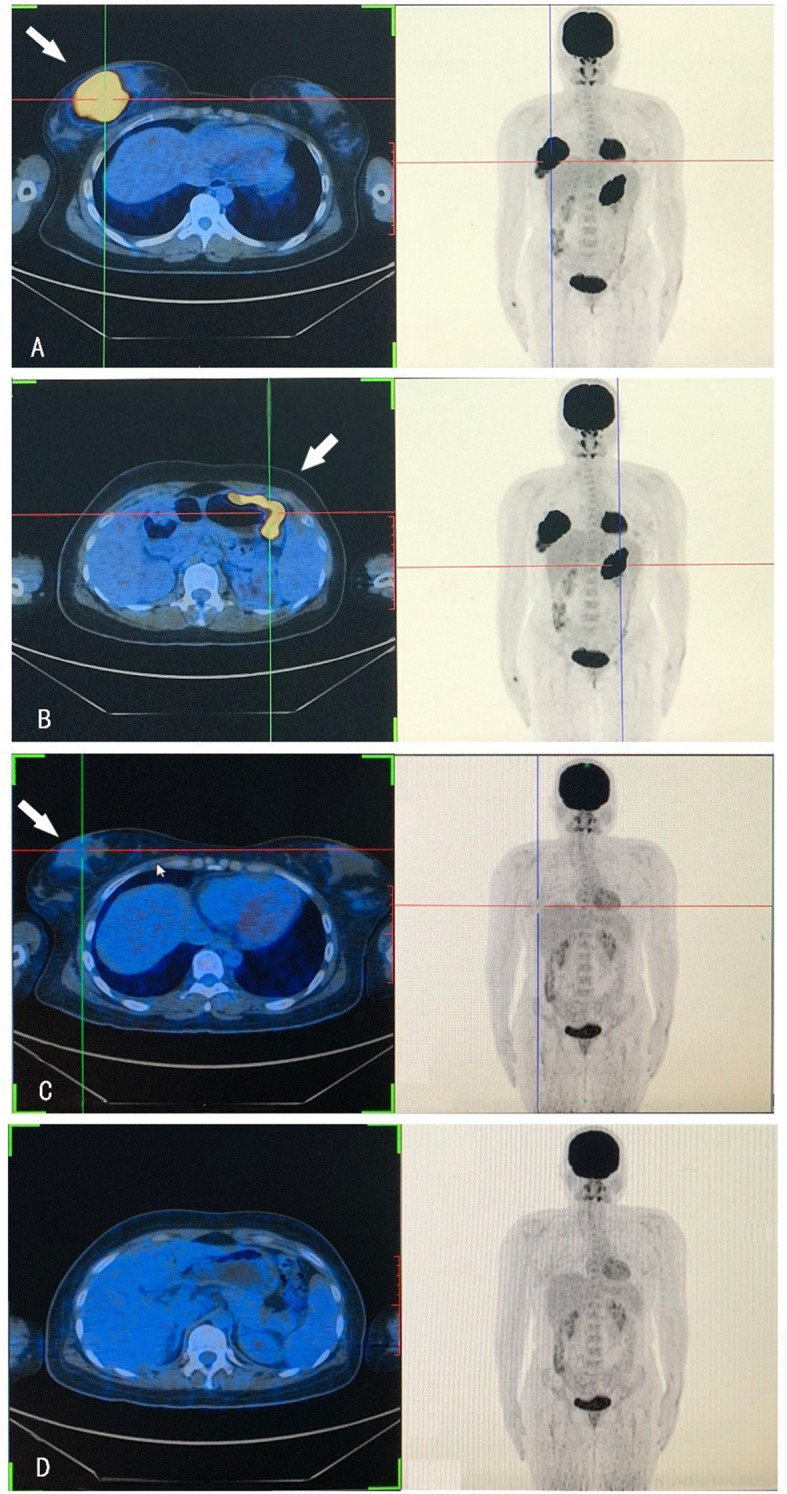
A whole-body positron emission tomography (PET)-CT scan revealed intense FDG uptake in the right breast (A) and greater curvature of the stomach (B). After four cycles of modified SMILE regimen, the right breast mass was significantly smaller than before (C). FDG uptake in the stomach wall disappeared (D)
